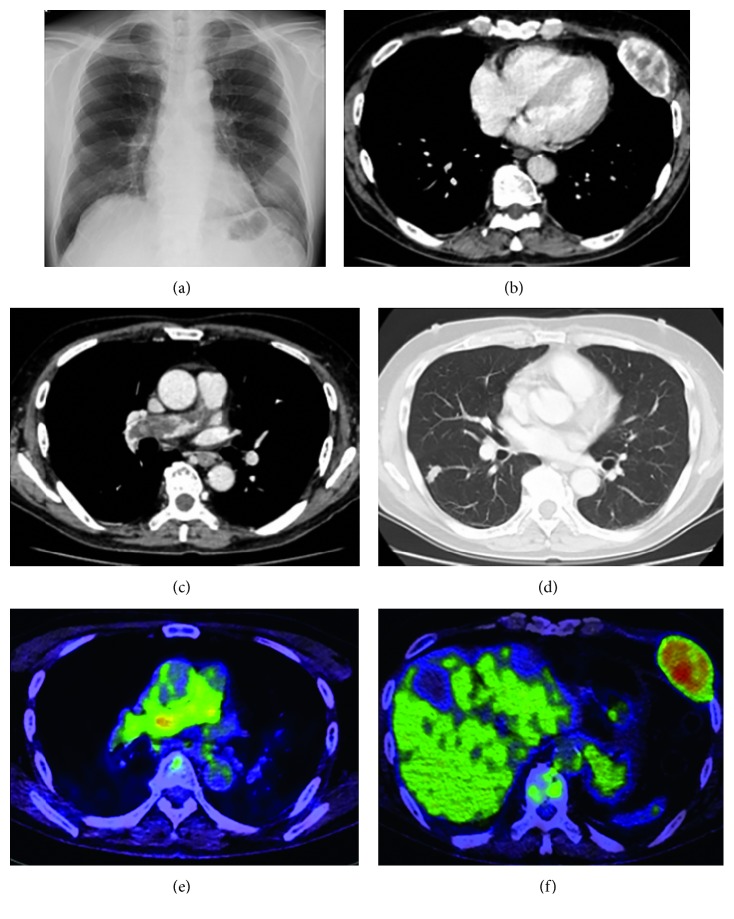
Radiological examinations at diagnosis. A chest radiograph showed a faint infiltrative shadow in the left lower lung field (a). Contrast medium-enhanced chest computed tomography showed a large tumor in the left anterior chest wall (b). An enhanced mass in the right pulmonary artery (c) and small nodules in the right lung (d) were also found. Fluorodeoxyglucose (FDG)-positron emission tomography revealed positive FDG uptake in the pulmonary artery mass (e) and in the chest wall tumor (f).
